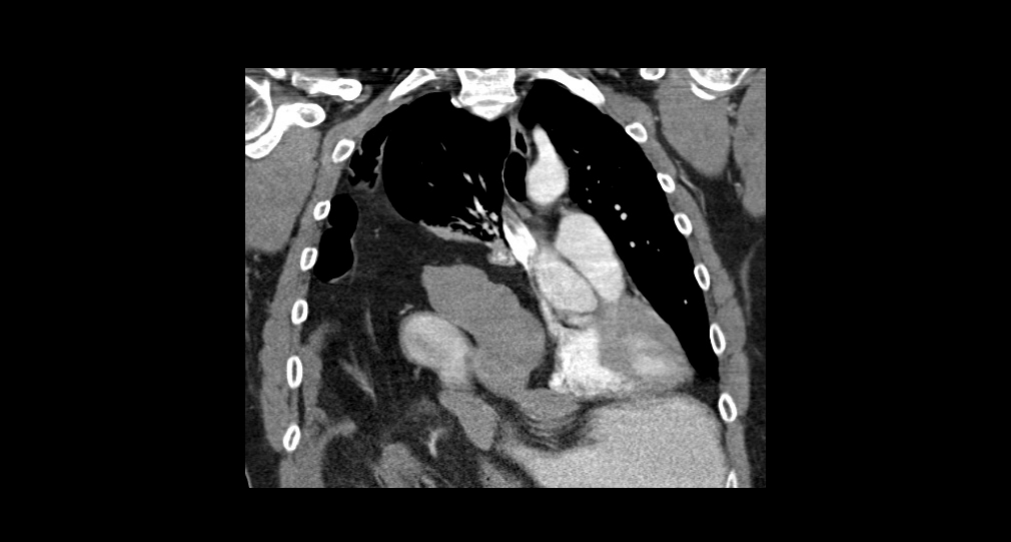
Coronal CT demonstrating herniated bowel and liver into right thorax.
